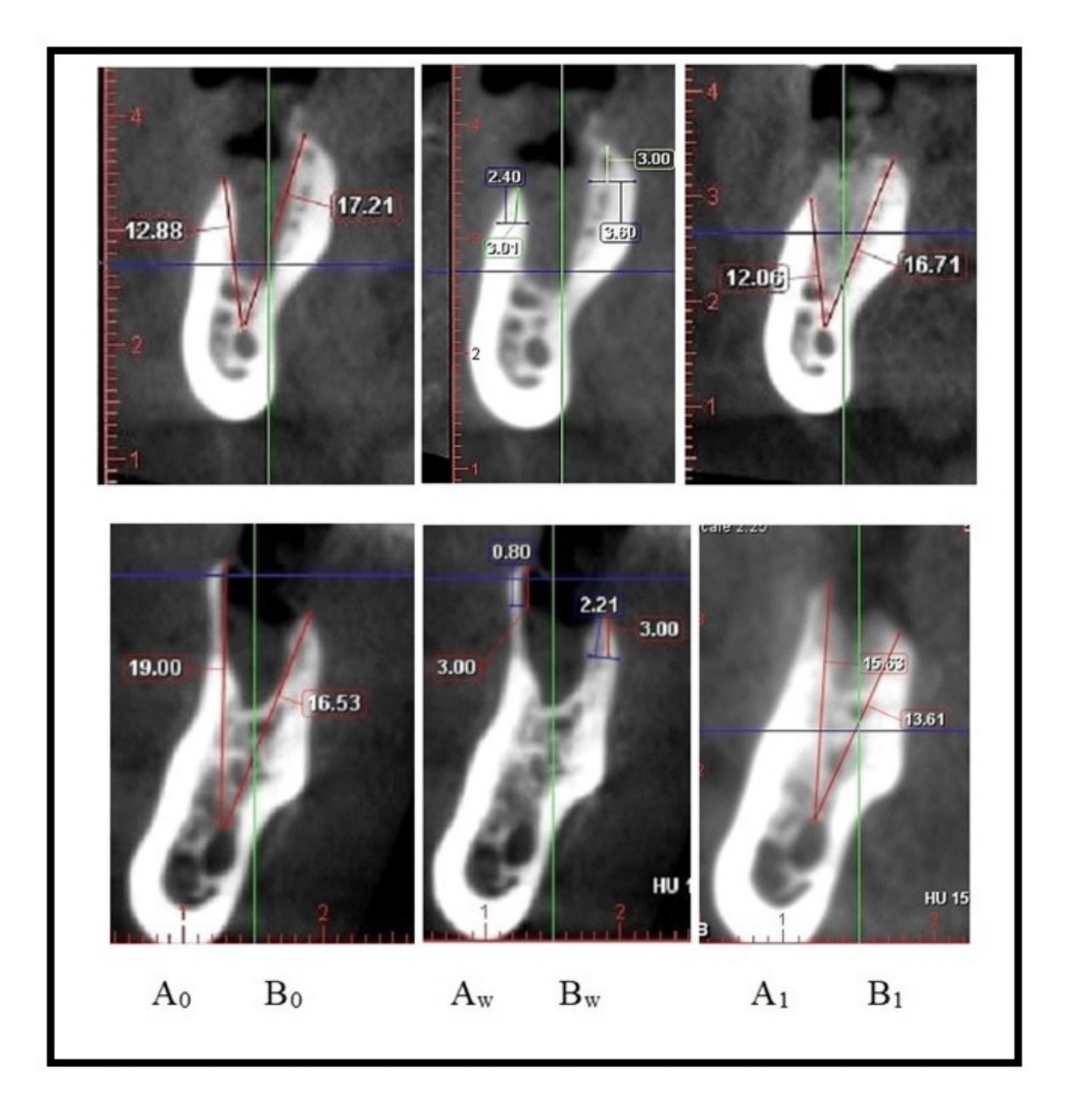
Cone-beam computed tomography images on the day of the extraction (left and middle) and three months after the surgery (right): paraxial slices A0: Buccal bone height on the day of extraction; B0: Palatal/lingual bone height on the day of extraction; Aw: Buccal bone width on the day of extraction; Bw: Palatal/lingual bone width on the day of extraction; A1: Buccal bone height three months after the extraction; B1: Palatal/lingual bone height three months after the extraction.
